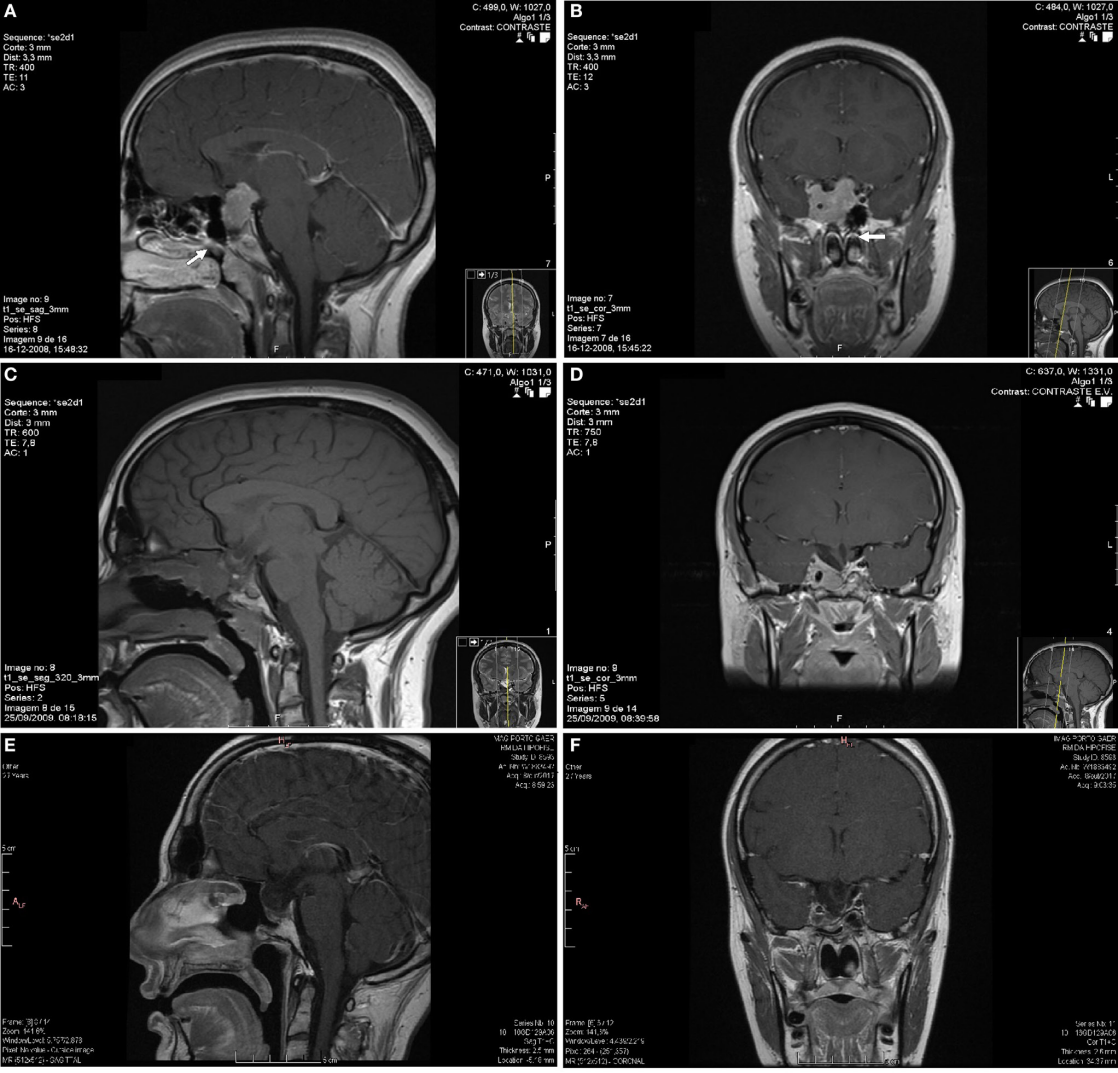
Sagittal (A) and coronal (B) plane of the first pituitary magnetic resonance imaging (MRI) with an invasive macroadenoma with gadolinium enhancement. The pituitary tumor measured 32 mm × 30 mm × 25.5 mm in anteroposterior, craniocaudal, and transverse dimensions, respectively. The lesion had invasion of the right cavernous sinus, superior deviation of the left internal carotid artery, sphenoid sinus invasion, and compression and stretching of the optical chiasm. The tumor was isossignal area in T1 and T2 with small cysts and had marked contrast enhancement. The possible nasal metastasis was localized near the sphenoid rostrum in the left nasal fossa (arrows). The postoperative MRI (C,D) revealed a persistent expansive pituitary lesion with right cavernous sinus invasion. The last pituitary MRI (E,F) performed in October 2017 showed a pituitary tumor with markedly reduced dimensions.
